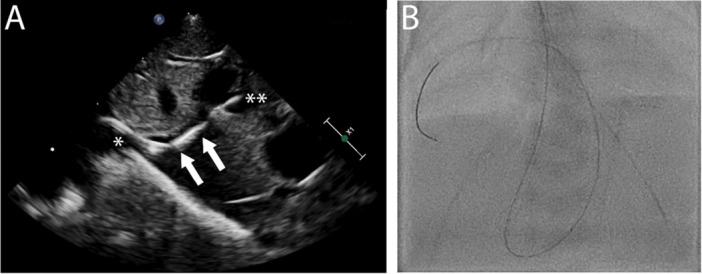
(A) Echocardiographically guided advancement (longitudinal view of the upper abdomen) of the wire from the inferior vena cava (IVC, *) toward the portal vein (PV, ), across the closed ductus venosus (DV, two arrows). (B) Fluoroscopic image demonstrating the final wire position following passage of the DV and PV via the descending vertical vein to the right inferior pulmonary vein. [Color figure can be viewed at wileyonlinelibrary.com]
